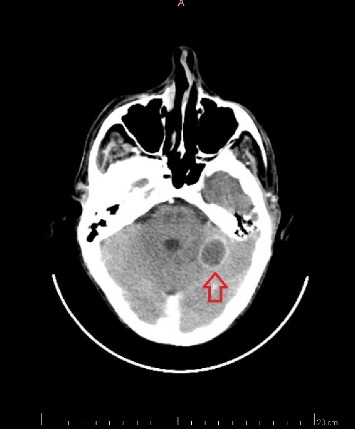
Axial CT scan of the brain showing a hypodense, rounded lesion in the left frontal gyrus (red arrow), consistent with a cerebral abscess. The lesion exhibits a central low-density area, indicative of necrotic or liquefied content, surrounded by a thin rim, which typically represents the capsule of the abscess.
